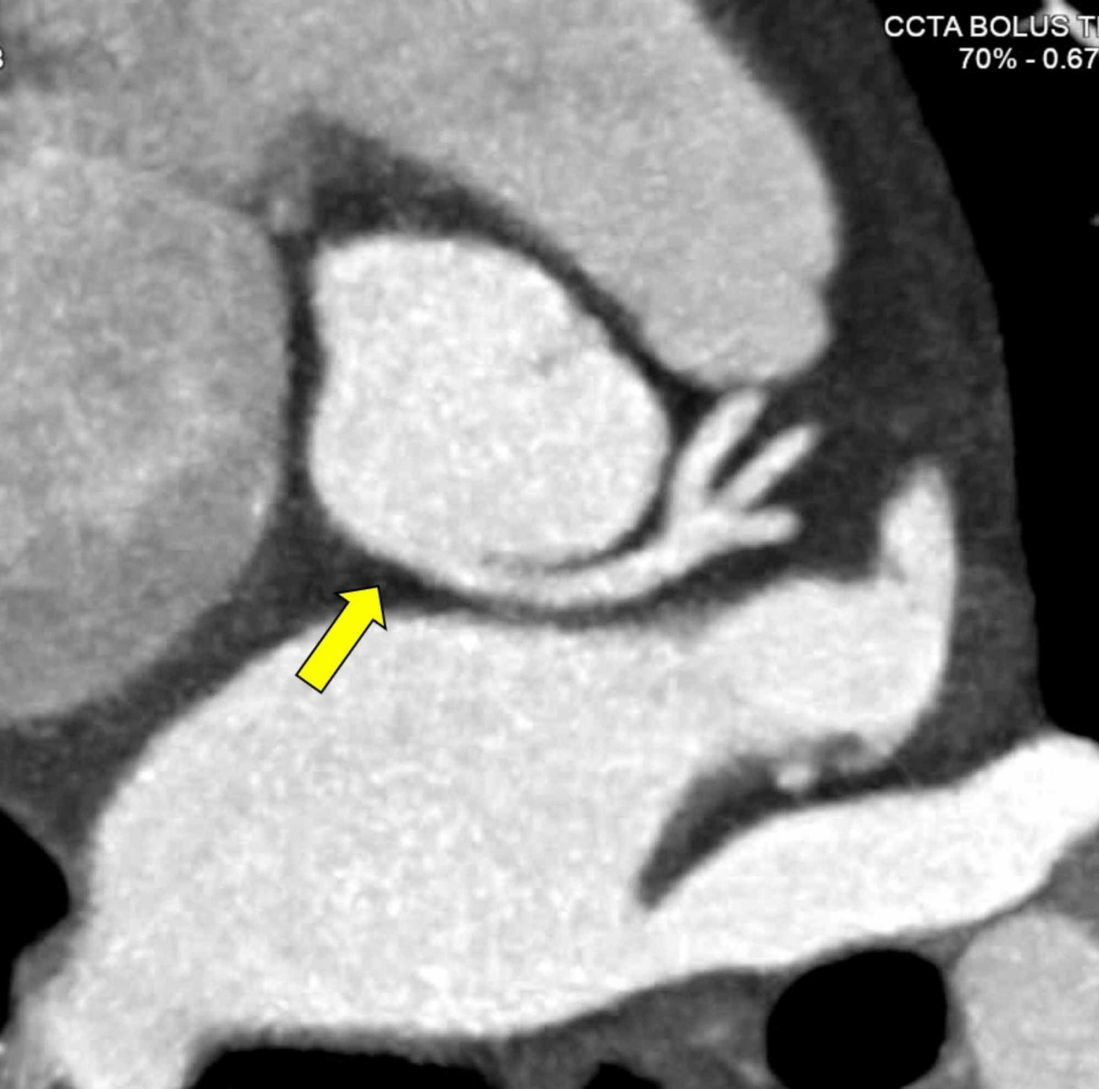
CCTA demonstrating LCANCC LCA with a retro-aortic course (arrow), trifurcating into the left anterior descending, ramus intermedius, and left circumflex arteries. CCTA: computed tomography coronary angiogram; LCANCC: left coronary artery from the non-coronary cusp; LCA: left coronary artery
